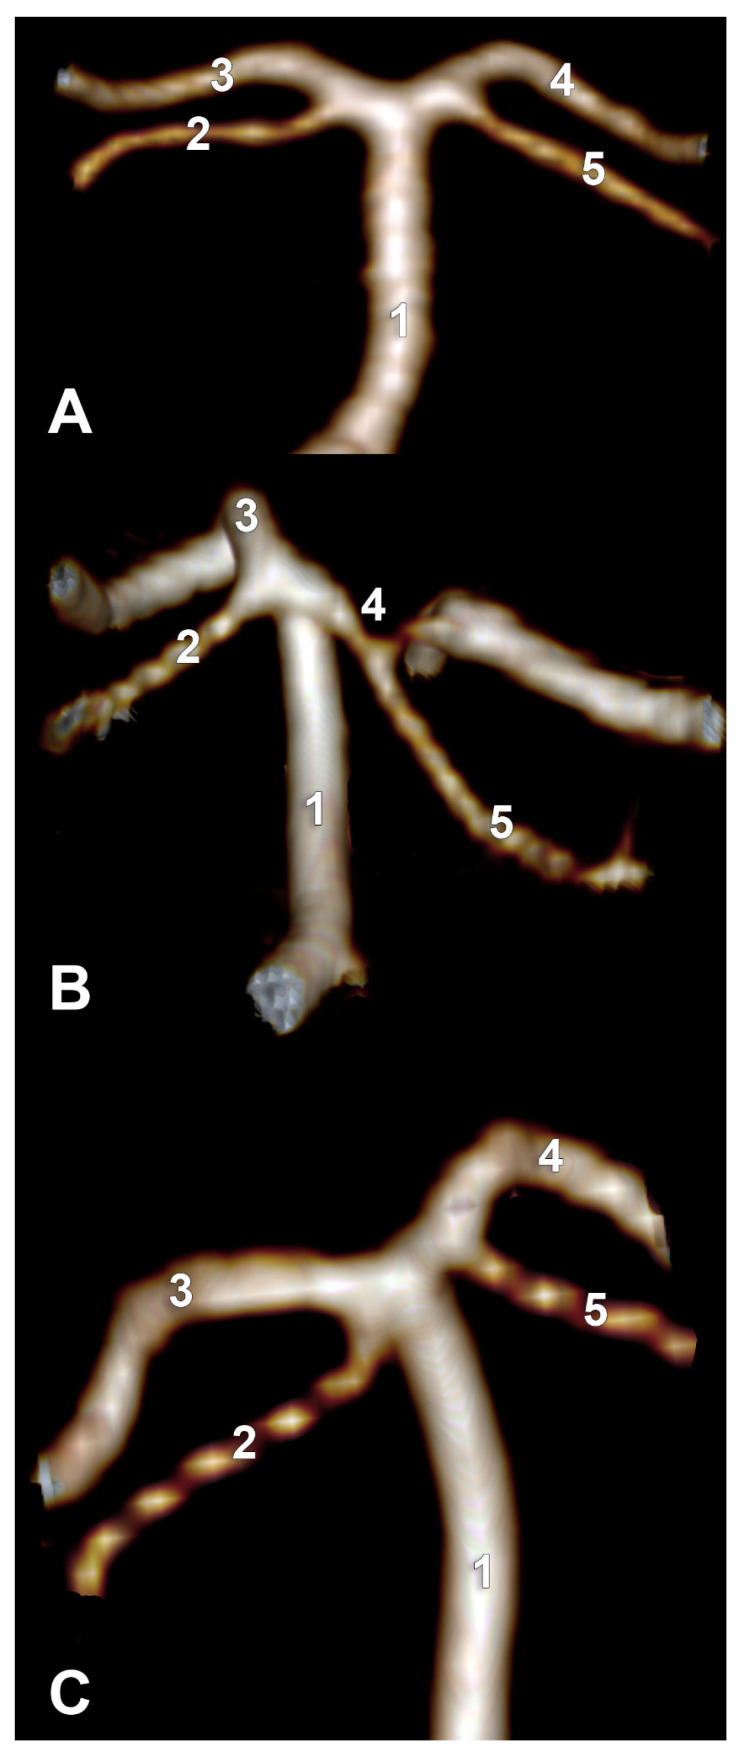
Type H of the bilateral combination of superior cerebellar arteries origins. Posterior views of basilar arteries. CT angiograms with three-dimensional volume renderings. (A) Bilaterally symmetrical PCA origins of SCAs. (B) The hypoplastic right P1 segment of PCA. (C) Bilaterally asymmetrical PCA origins of SCAs. 1. BA; 2. left SCA; 3. P1 segment of left PCA; 4. P1 segment of right PCA; 5. right SCA.
